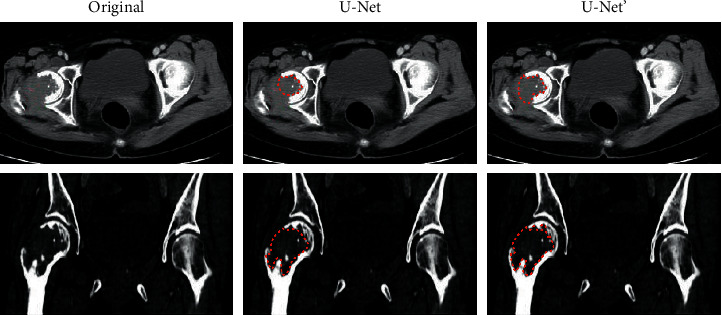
Segmentation of CT images for multiple myeloma.
